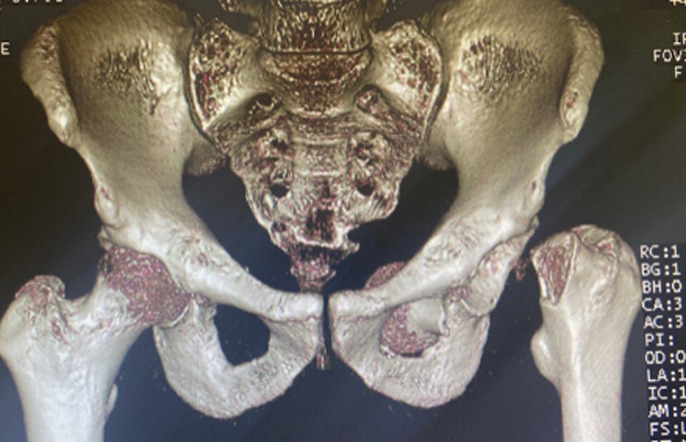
three-dimensional computed tomography image showing the femoral head in an intrapelvic position
